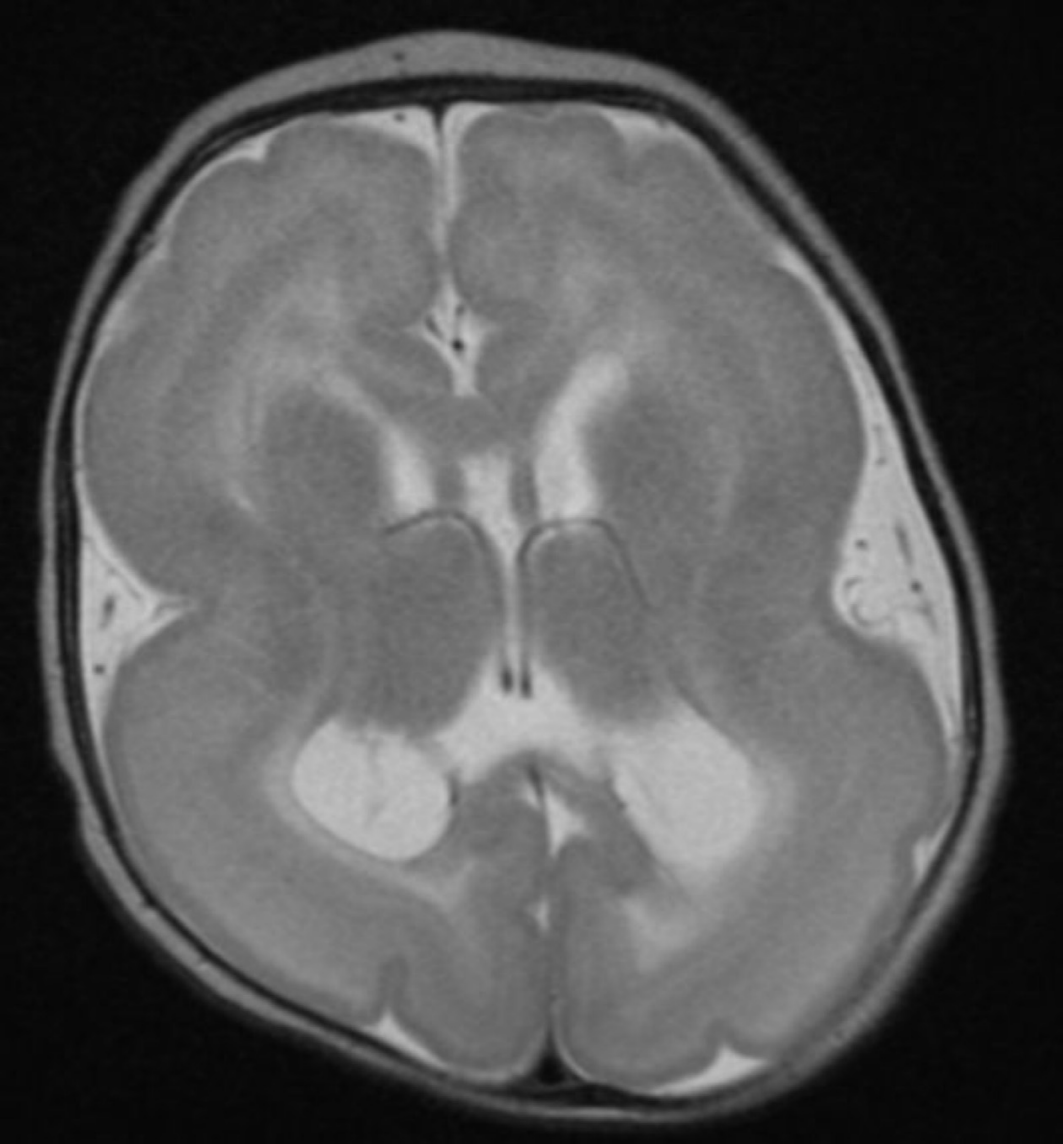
MRI of the brain reveals lissencephaly. Shown is a selected image from an axial T2‐weighted MRI scan of the brain performed at 2 months 1 week of age. There is a paucity of cortical sulcation with broad, thickened gyri, and a near‐smooth and thickened appearance of the surface of the cerebral cortex, perhaps with a slight posterior predominance. The brain exhibits the typical “hourglass” configuration of lissencephaly. T2‐weighted scans further reveal a hyperintense, cell‐sparse zone within the cerebral cortex further characteristic of lissencephaly. The corpus callosum is dysplastic.
